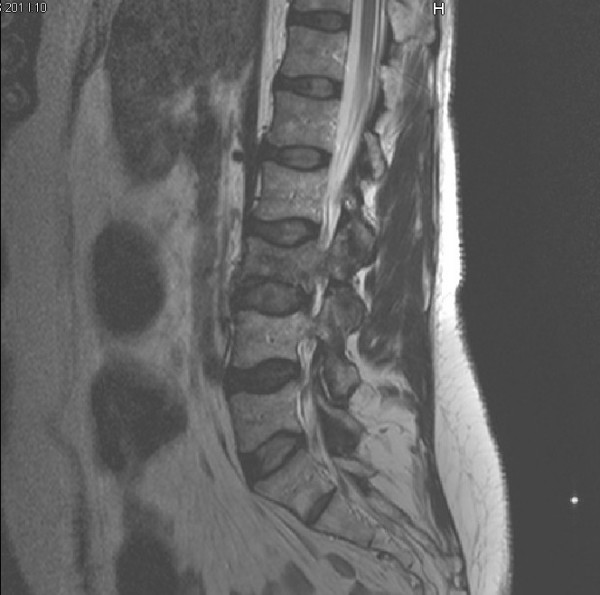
Sagittal T1-weighted lumbar Spine MRI for patient #1. There is low signal intensity destruction within the vertebral body extending into the right neural arch at the L3 level. In addition, collapse of the L3 vertebral body is identified.
